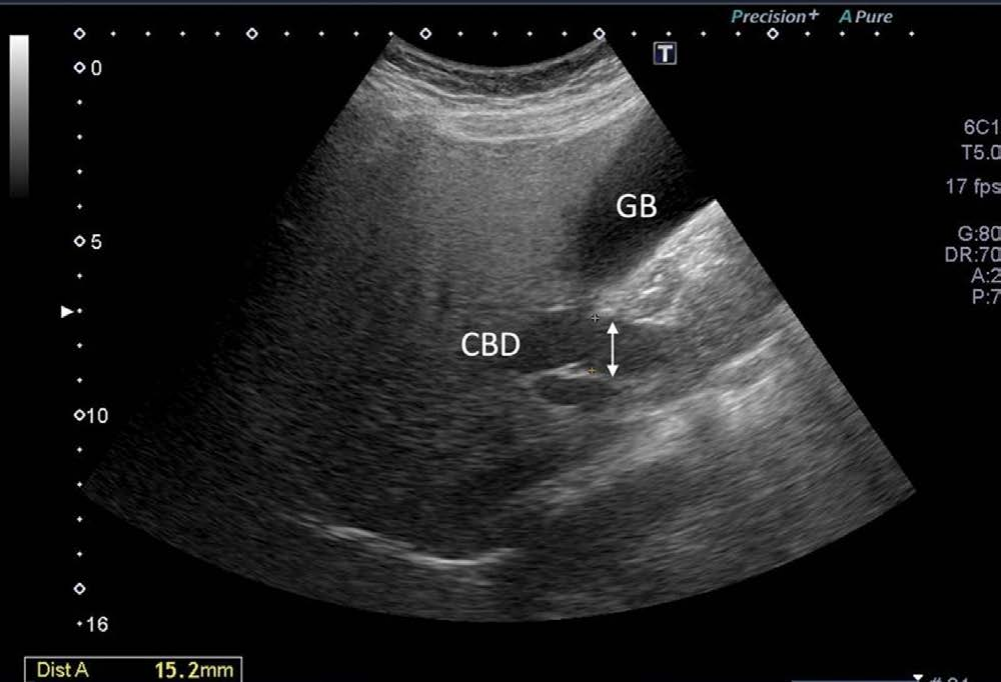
Abdominal ultrasonography showed dilated common bile duct up to 15.2 mm without obvious stones nor mass.
